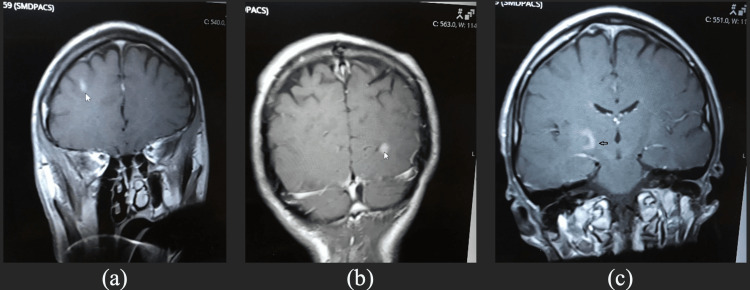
MRI of the brain with T2-weighted images (a) Hyperintensive lesion in the right frontal lobe. (b) Left occipital lobe lesion. (c) A thin rim enhancement in the medial right of the internal capsule lesion.
